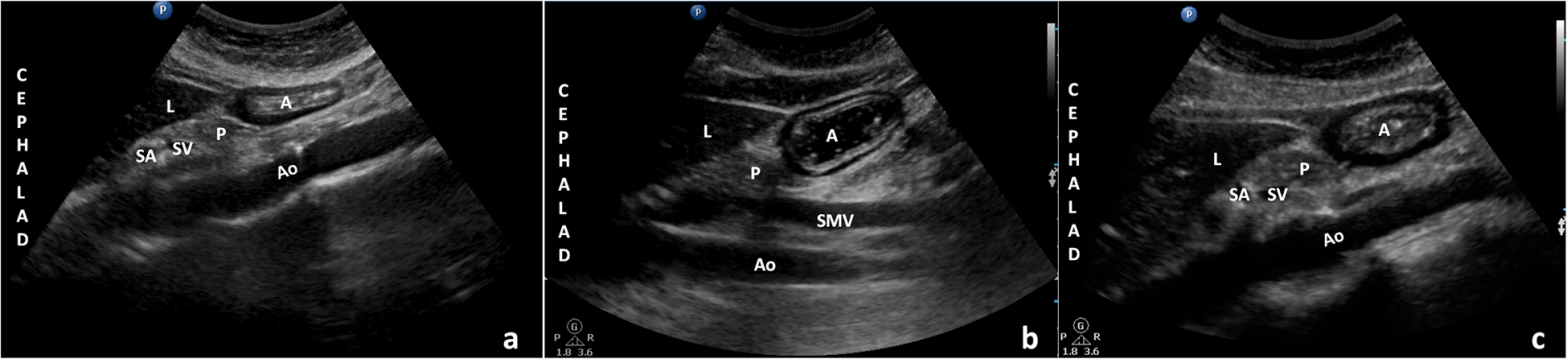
Sonographic image of the gastric antrum in the semi-recumbent position. Ultrasound examinations were conducted with a low-frequency curvilinear array probe from a Philips (CX50), showing an empty gastric antrum (a), liquid (b) and semi-solid (c) food in the gastric antrum. A sagittal cross-section of the antrum in a plane, including the left lobe of the liver anteriorly, the pancreas and aorta posteriorly was acquired. A, antrum; L, liver; P, pancreas; SA, splenic artery; SV, splenic vein; Ao, aorta; SMV, superior mesenteric vein
